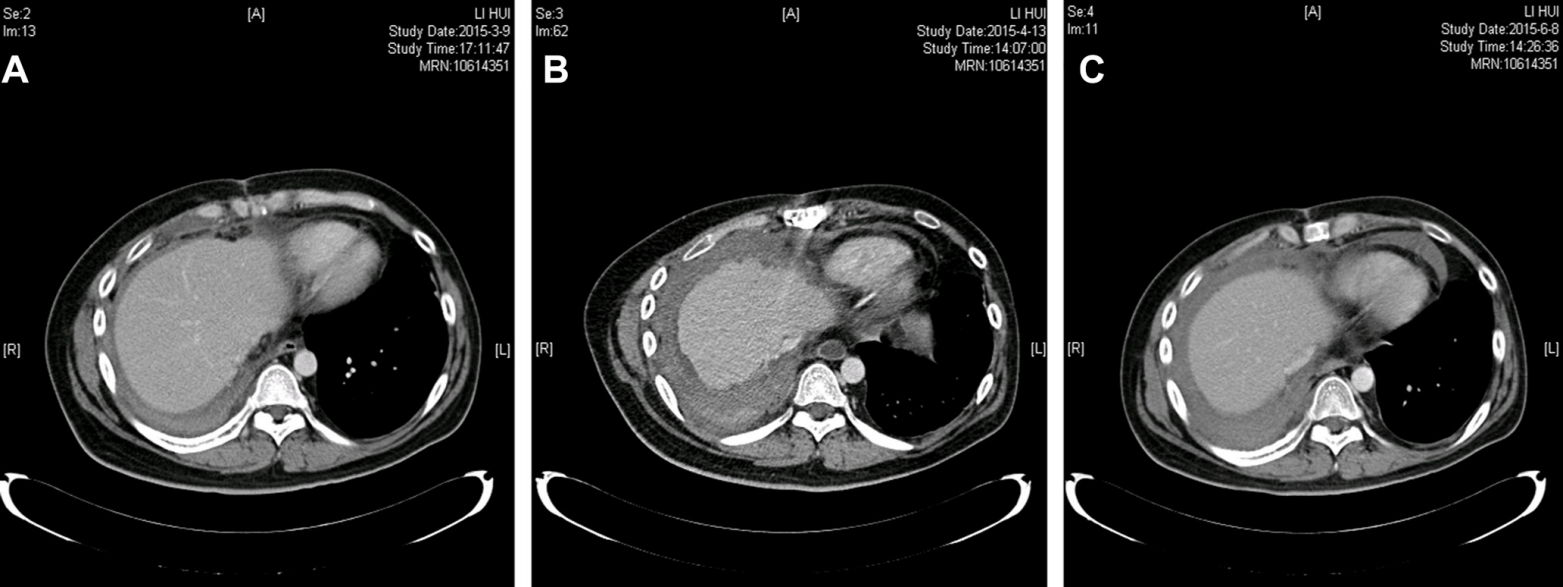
Contrast-enhanced computed tomography (CT) scan of the abdomen (A) Peritoneal effusion with multiple peritoneal metastasis. (B) Peritoneal metastasis became progressive after one cycle of BEP regimen. (C) Radiologically stable disease was achieved after FORFIRI regimen.
